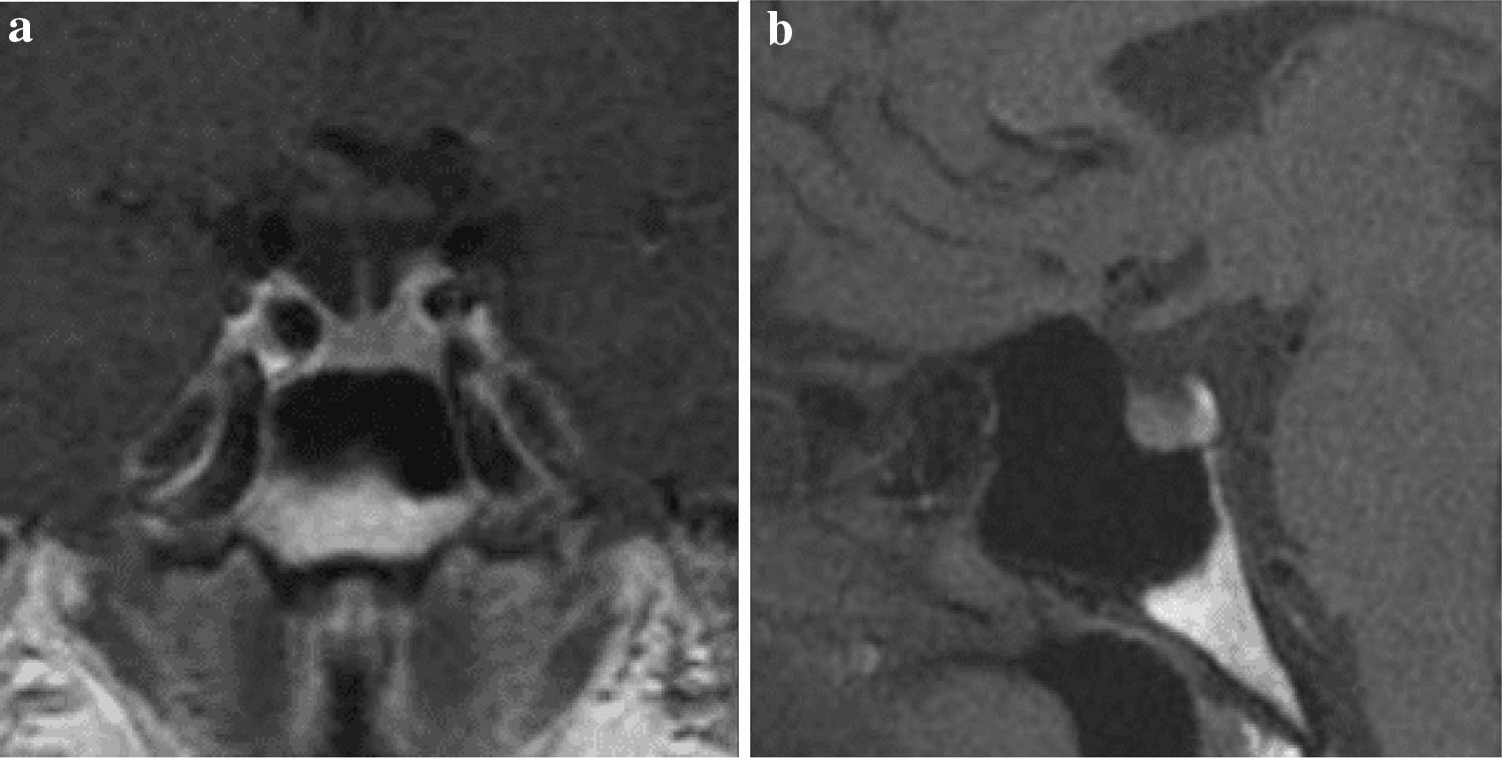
Targeted MRI examination of the pituitary region including coronal and sagittal T1-weighted sequence post-contrast enhanced images revealing no abnormalities
